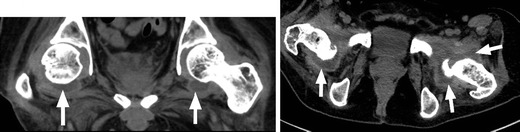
Benign hip effusions. Large bilateral hip effusions (*white arrows*) detected incidentally on CT abdomen and pelvis obtained for another indication. These were found to be sterile effusions in this patient without fever or elevated white blood cell count
